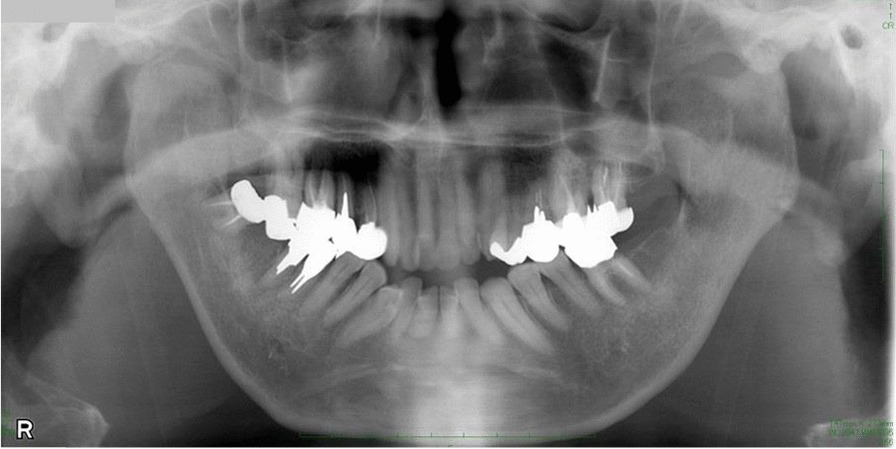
Postoperative panoramic radiographs of the patient. No temporomandibular joint dislocation recurrence
